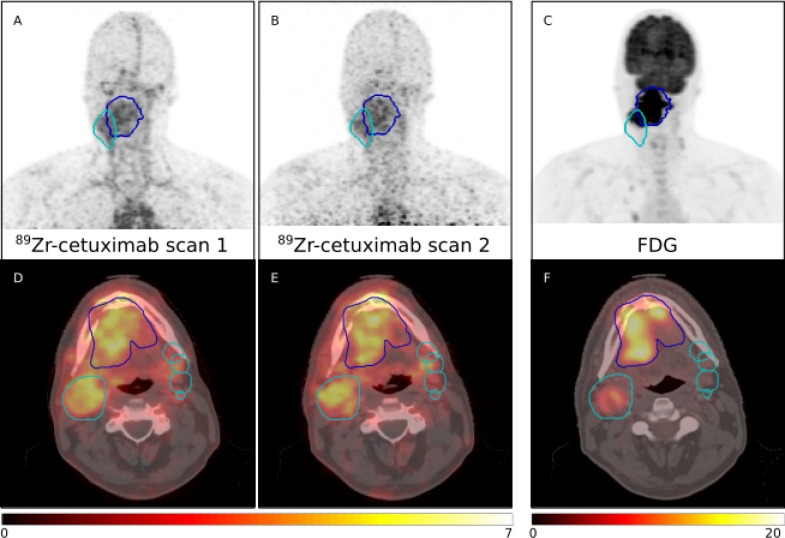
PET maximum intensity projections (MIP) (top row) and fused PET/CT images showing PET uptake in SUV (bottom row) of the two ^89^Zr-cetuximab scans and FDG PET/CT of one patient (patient 6) The GTV for the primary tumor is depicted in blue; the CTV for the lymph nodes in cyan. Only the largest lymph node is displayed in the MIP.
